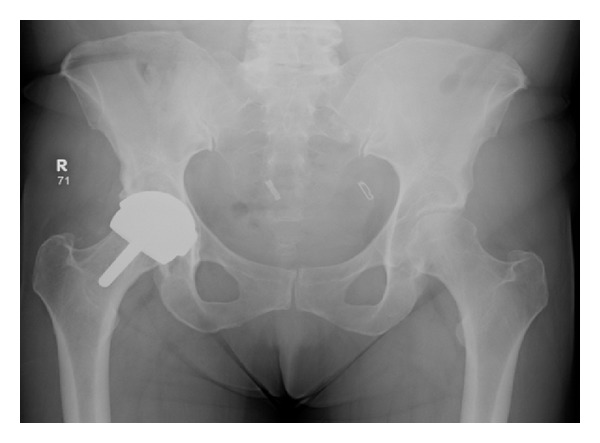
Pelvis radiograph from 2009.
